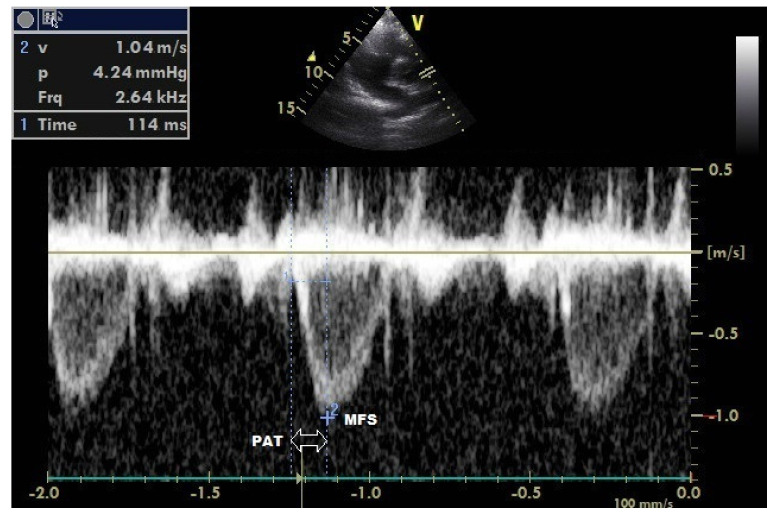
Evaluation of pulmonary arterial stiffness (PAS) by echocardiography. PAS (kHz/ms) = maximum frequency shift (MFS)/pulmonary flow acceleration time (PAT).
